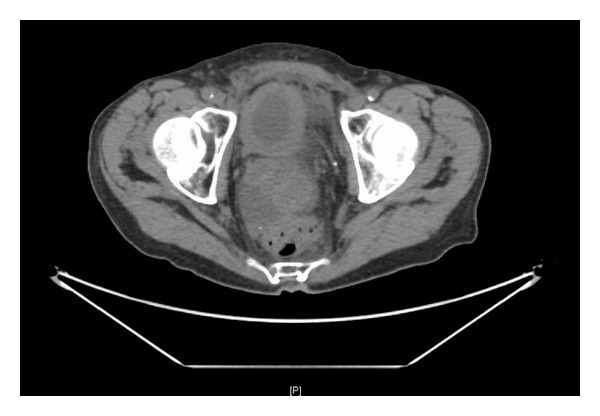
Pelvic CT showing diffuesly thickened bladder wall.
